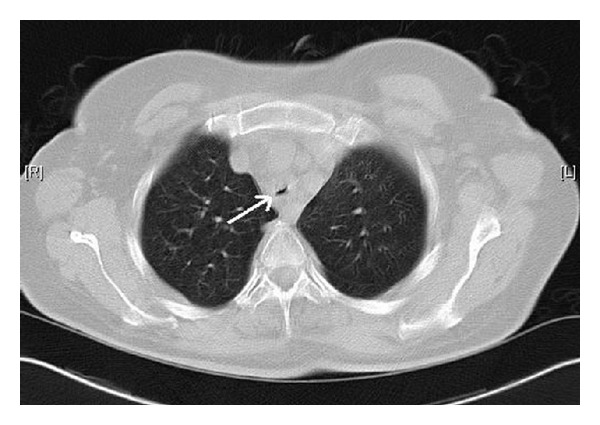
CT scan revealing tracheal stenosis.
